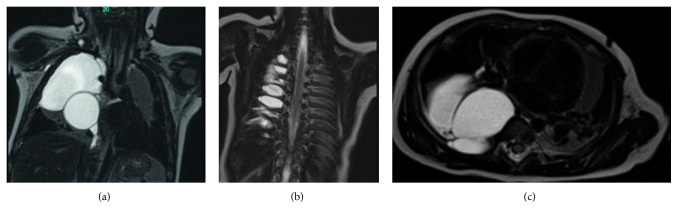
Magnetic resonance imaging (MRI): insinuating multiloculated cystic lesion. (a) Small extrapleural extensions of the cystic mass superiorly and laterally. (b) The mass bulges in the posterior intercostal spaces, without neuroforaminal involvement. (c) Posterior intercostal bulging, with no neuroforaminal involvement, and hilar structures are not compressed.
